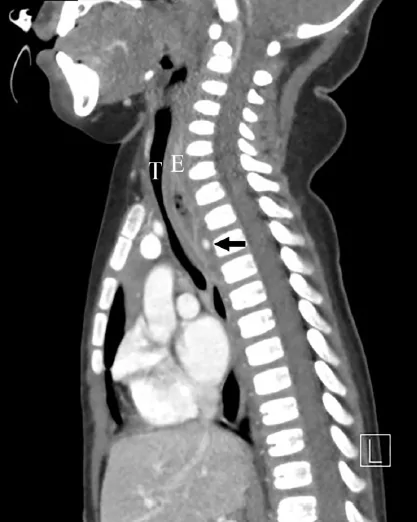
Sagittal contrast-enhanced computed tomography image shows tracheal stenosis (T) due to a dilated esophagus (E) that had expanded because of food residue accumulation above the esophagus segment, which is compressed by an aberrant right subclavian artery (arrow).
